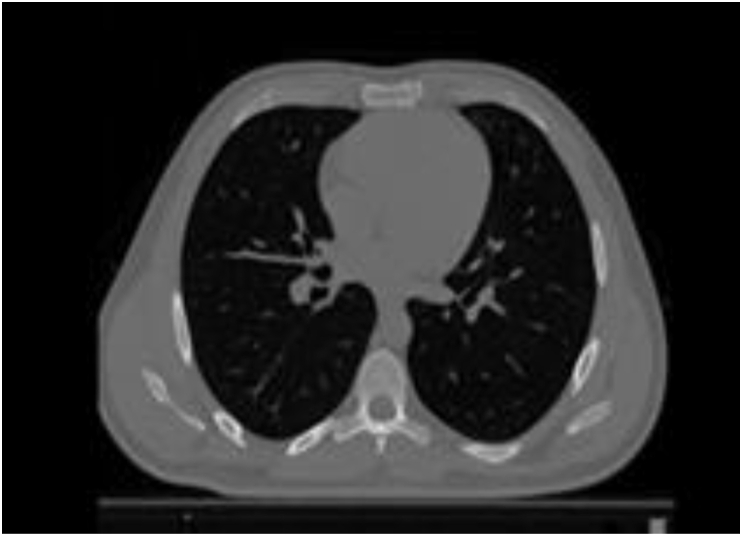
Chest CT slice of patient (A1) with no abnormalities.
